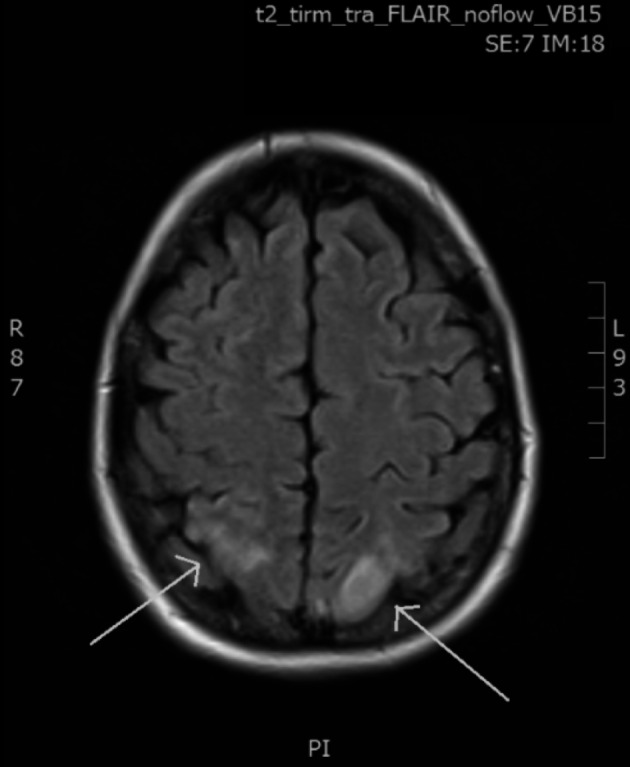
MRI performed during episode of blindness: posterior lesions in both occipital poles with a hyperintense signal (green arrow) on FLAIR sequence.
